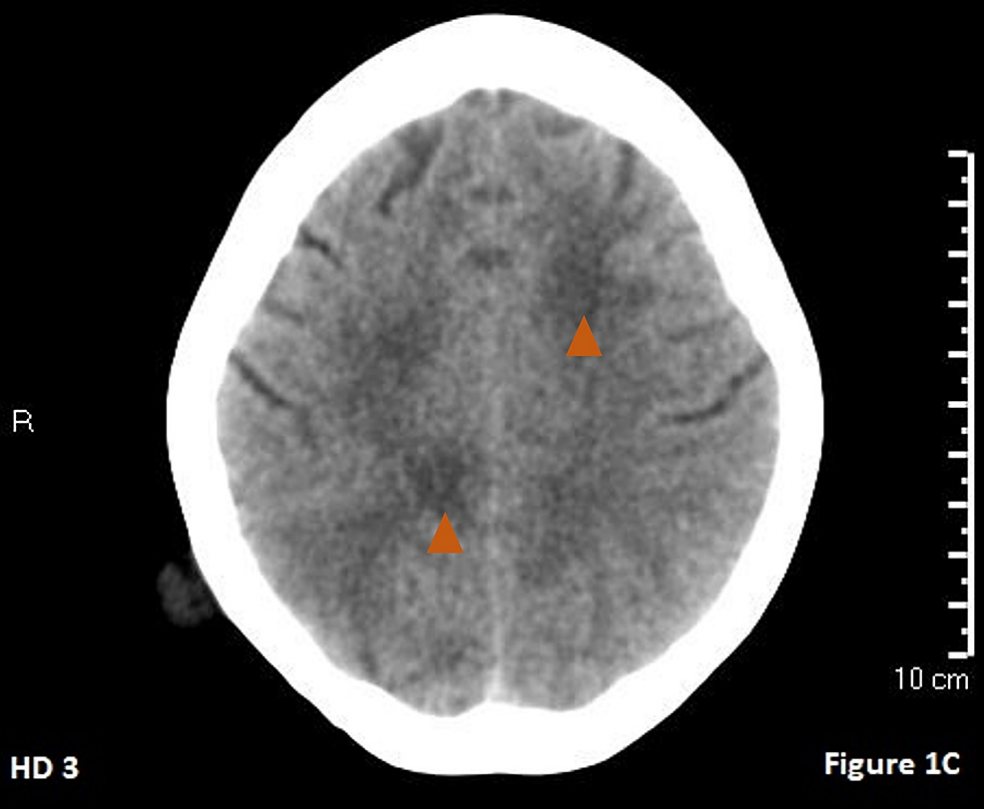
Hospital day 3 CT of the head. The orange arrows show slow progression of bilateral cerebral edema in comparison to hospital day 2.
